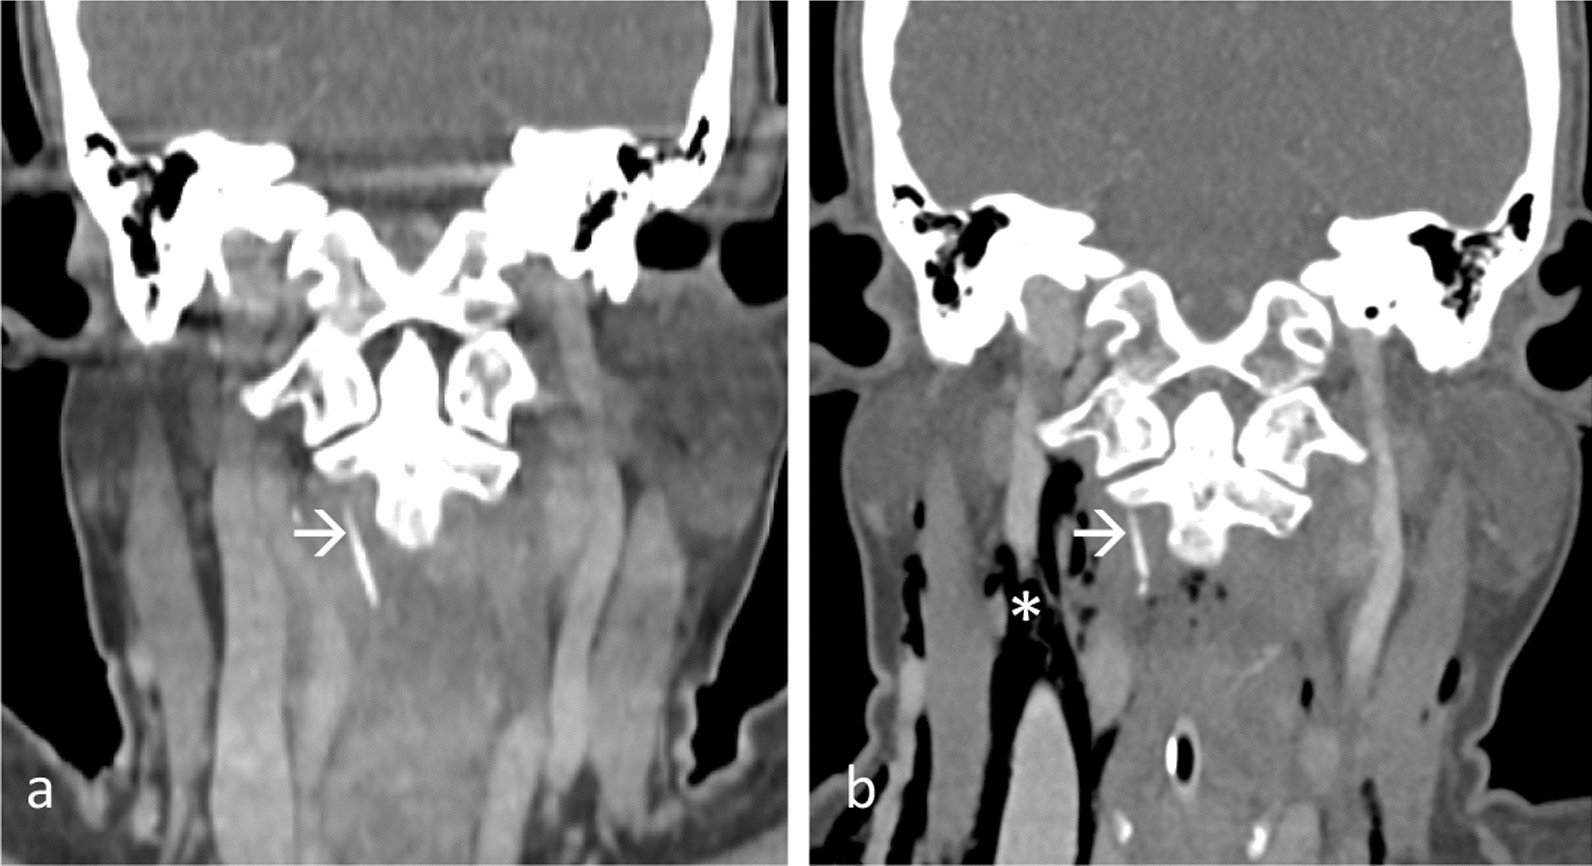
Contrast-enhanced coronal multiplanar reconstruction images a showed a linear fish bone (arrow) at right prevertebral region. Repeat CT b revealed the fish bone (arrow) at the similar region but broken into segments, complicated with pneumomediastinum (asterisk)
